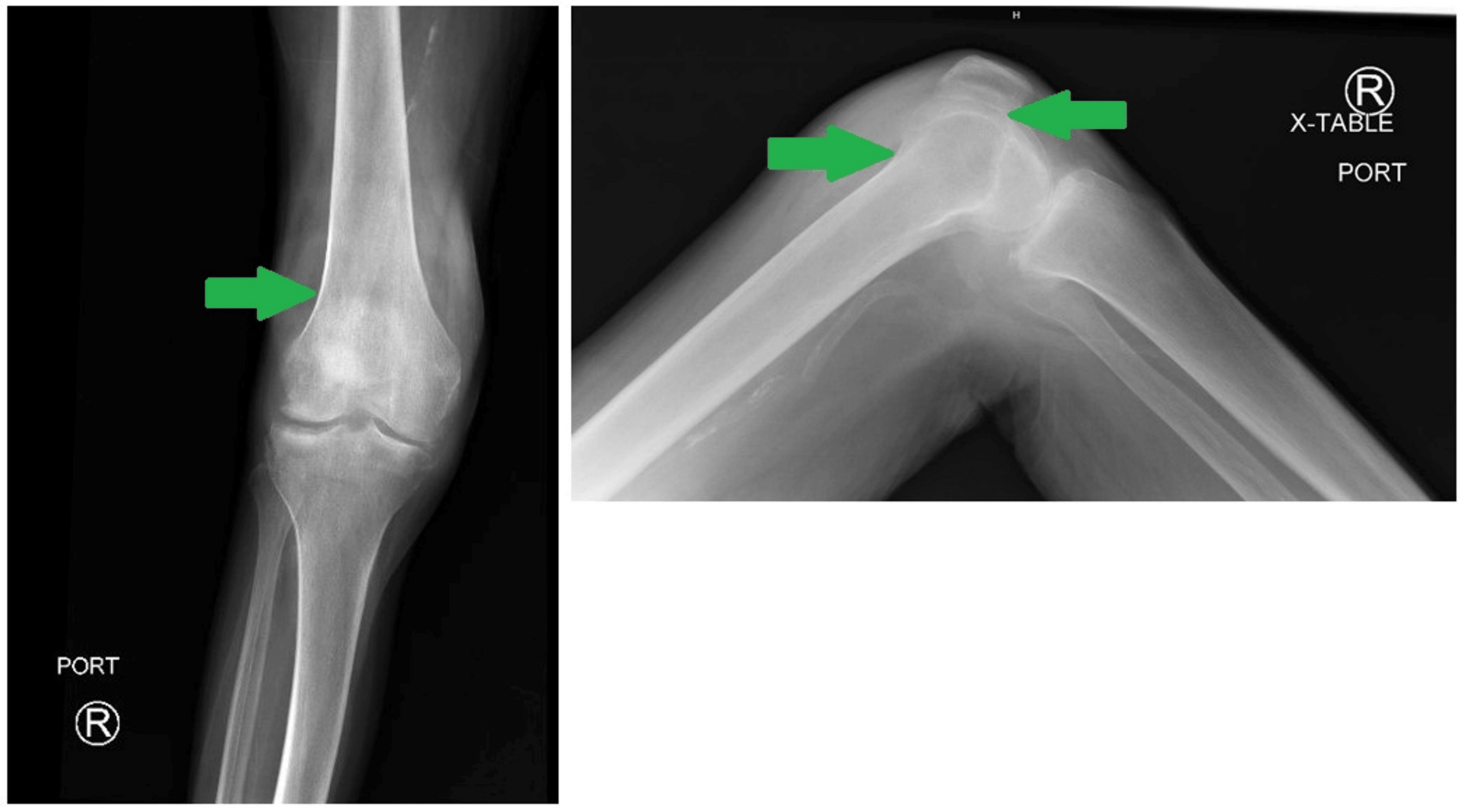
X-ray of right knee indicating joint effusion (green arrows)
